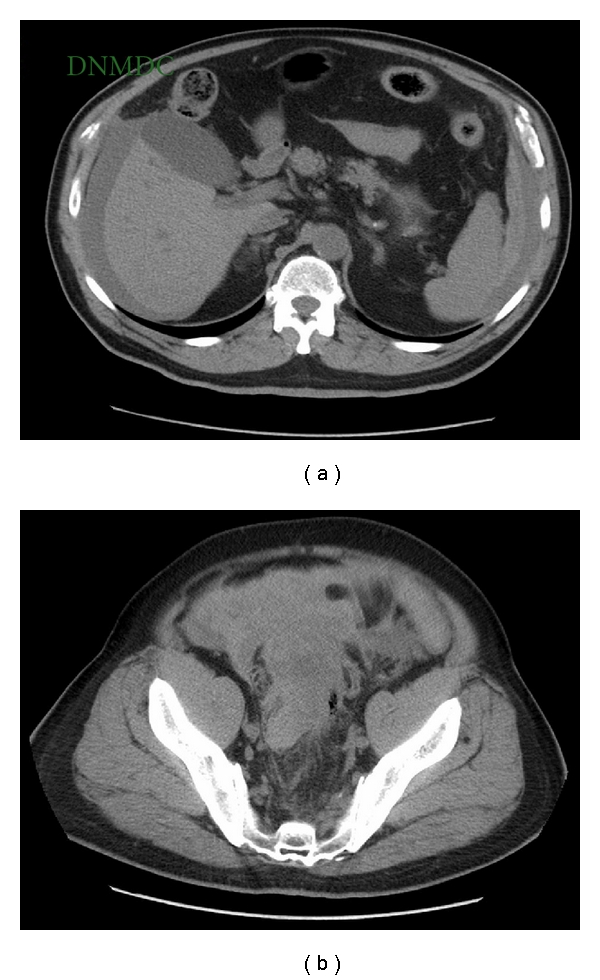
Preoperative computed tomography scan of the abdomen showing extensive hemoperitoneum (a) below the spleen and liver, among the small bowel, (b) in the left and right paracolic gutter, in front of the urinary bladder with a distended rectum.
